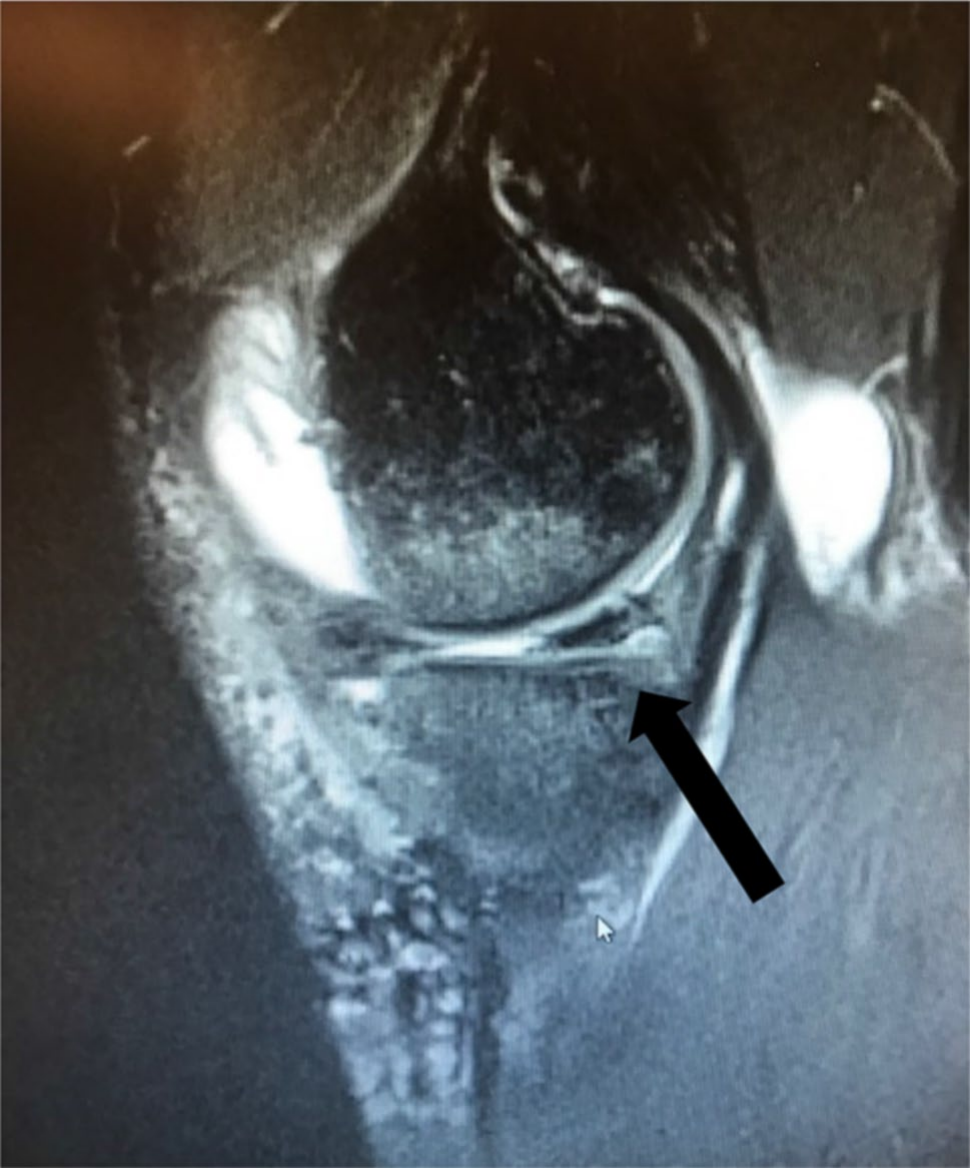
Sagittal view of MRI T2-scan showing the posterior horn of a medial meniscus transplant (arrow)
